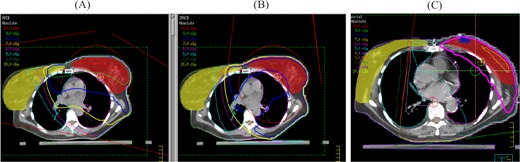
The two‐dimensional absolute dose distribution of a central slice is evaluated in three different beam‐delivery methods with 6 MUs. (A) Megavoltage cone‐beam computed tomography images with 200 projections; (B) megavoltage cone‐beam computed tomography images with 360 projections (from 0 degrees to 359.9 degrees in a clockwise direction); (C) orthogonal pair images.
